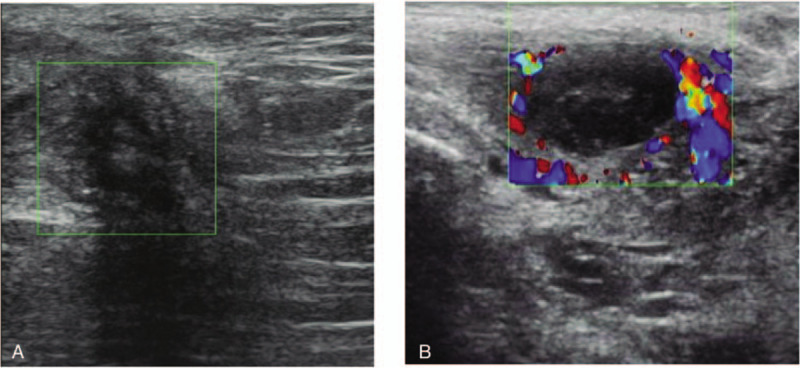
The typical ultrasonographic images of perineal endometriosis patients. The presentation of perineal endometriosis on ultrasound examination could be irregular hypoechoic without blood flow signal around (A), or a cyst with clear border and vascular signals around (B).
